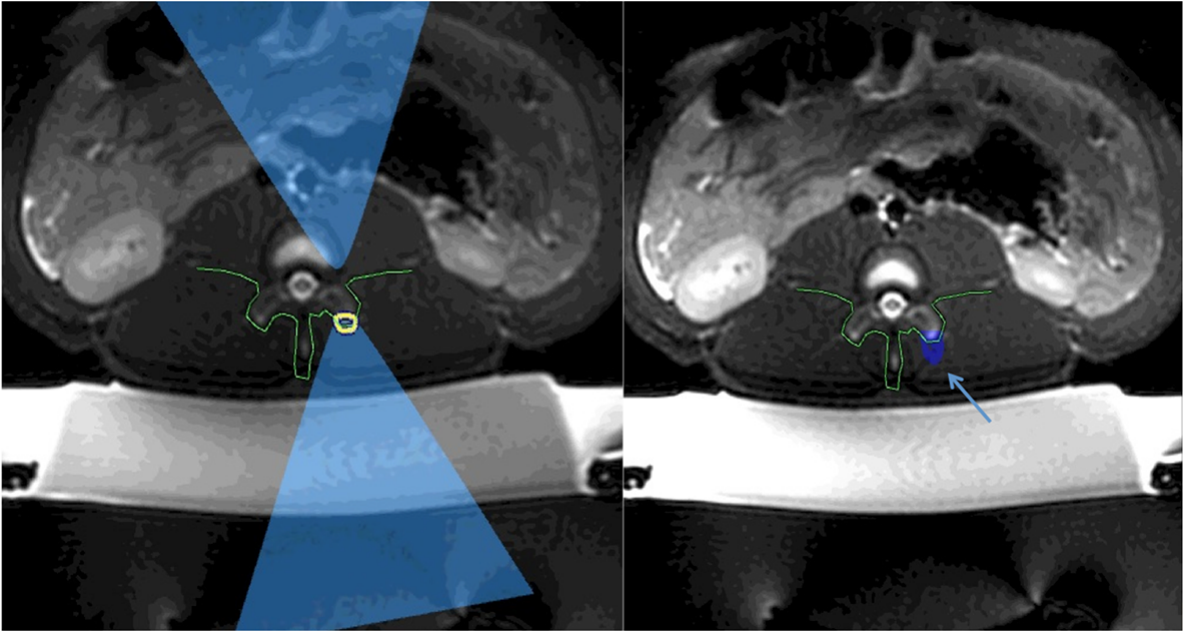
T2-weighted planning images are shown with the focal spot on the facet joint (*left image*). The beam orientation was chosen to best protect the spinal nerve roots, the spinal canal, and the spinal process as outlined in *green*. After HIFU treatment, the area of ablation is shown in *blue* (dose overlay) on the right image
